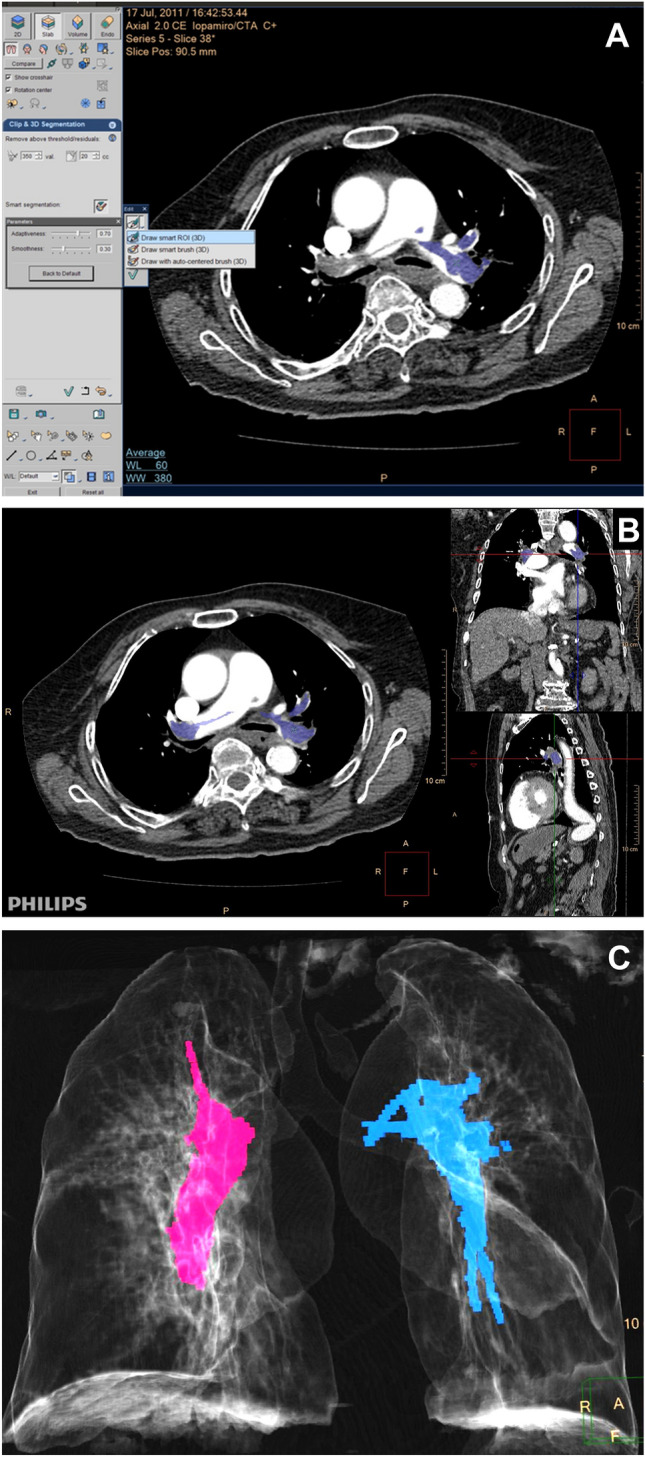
(A) 3D segmentation method (Draw smart ROI) was used to semi-automatically measure the volume of thrombus. (B) The boundaries of thrombus was checked visually on axial, coronal, and sagittal planes. (C) A 75-year-old female with high D-dimer (7898 mg/l) and mild elevated Troponin-I (0.64 ng/ml). 3D CT image quantification revealed large emboli burden in right pulmonary artery (14.3 cm^3^, pink color) and left pulmonary artery (15.03 cm^3^, blue color).
